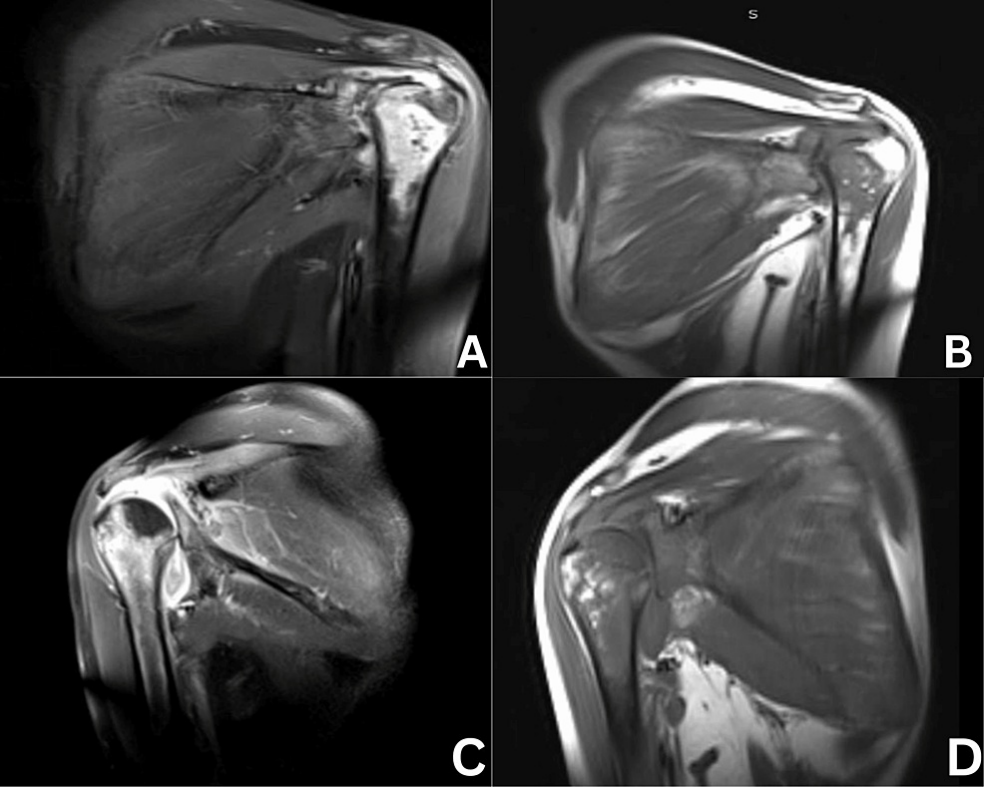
MRI of the patient T1W coronal images showing A: (right shoulder joint) hypointense area involving the cortex and medullary cavity of the head and proximal shaft of right humerus; B: post-contrast enhancement with minimal fluid intensity collection noted in the subacromial subdeltoid bursa and axillary recess; C: (left shoulder joint) hypointense area involving the cortex and medullary cavity of the head and proximal shaft of left humerus; D: post-contrast enhancement with minimal fluid intensity collection noted in the left glenohumeral joint MRI: magnetic resonance imaging
